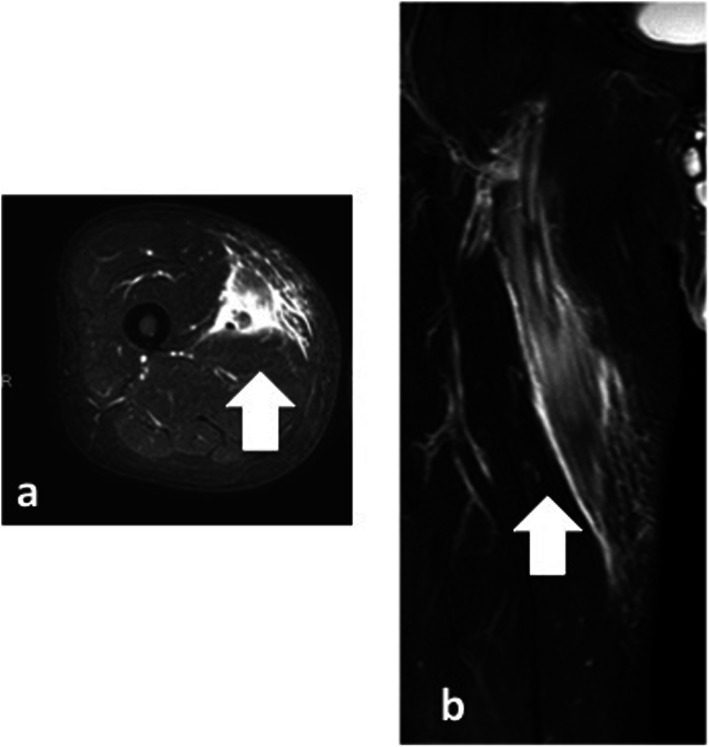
T2-weighted magnetic resonance images of the patient’s thigh. T2-weighted images (a, axial; b, coronal) revealed perivascular inflammation (arrows: edema of soft tissue) surrounding the superficial femoral artery
